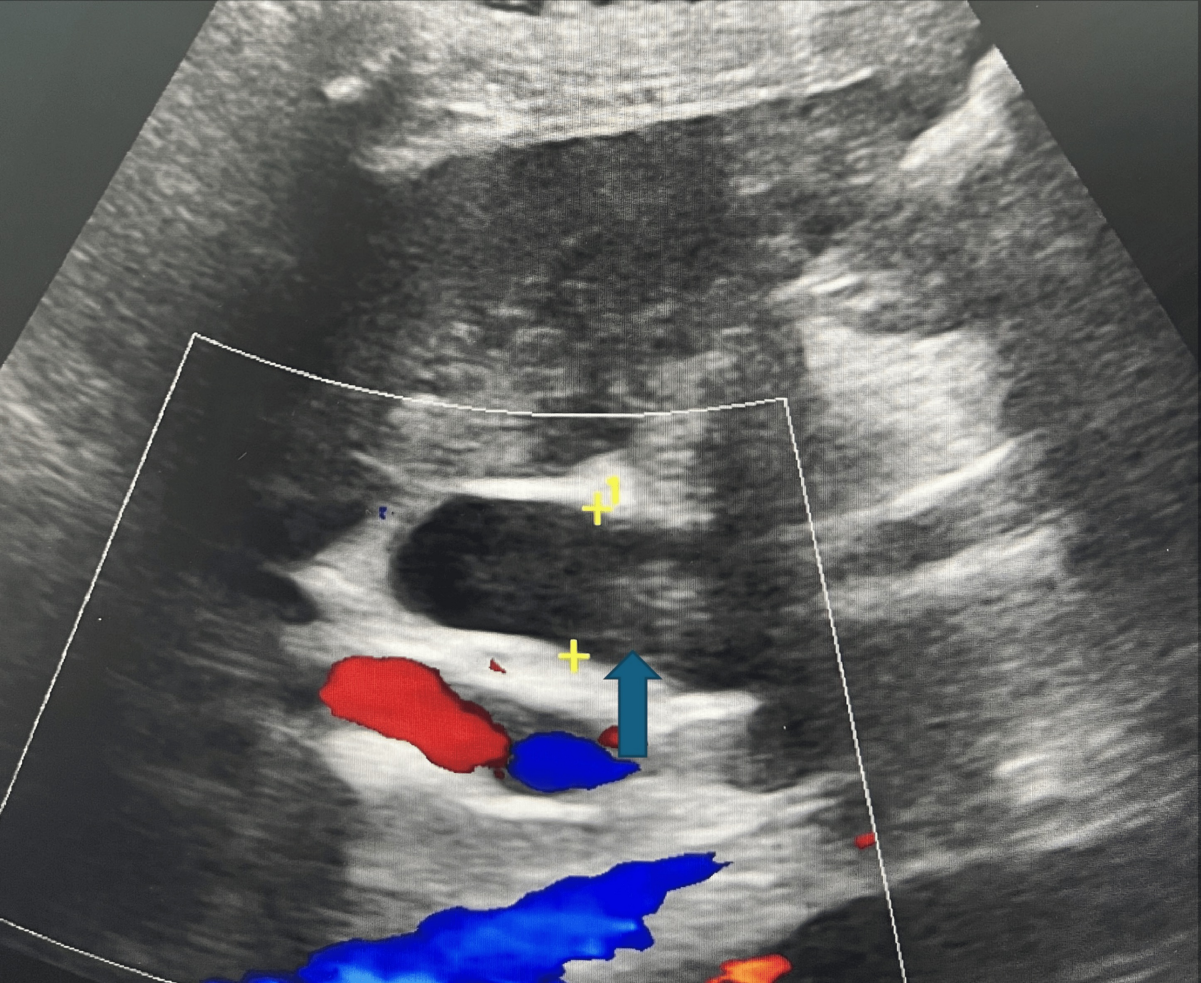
Ultrasonographic image showing a dilated common bile duct.
